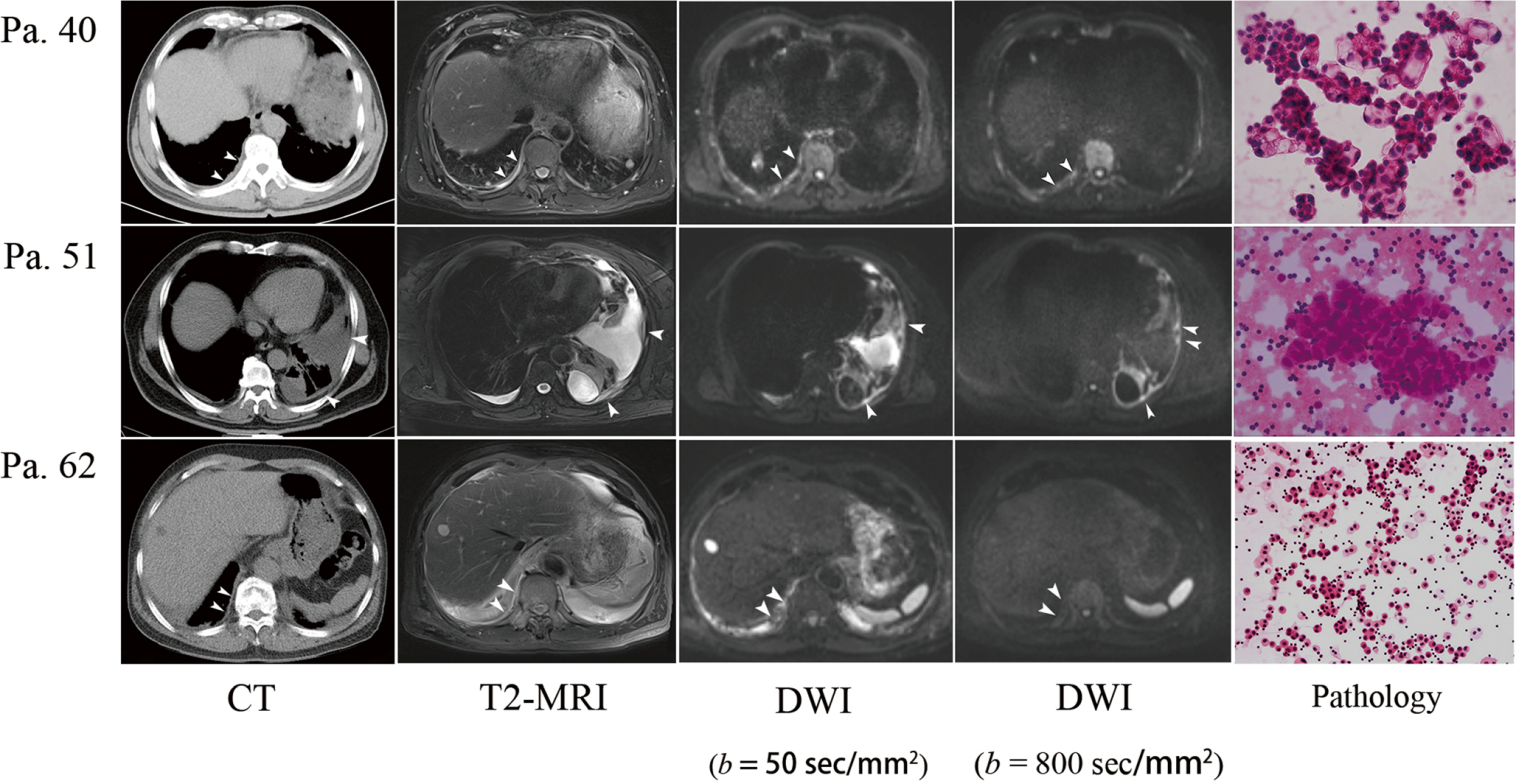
Representative cases. Patient 40 was a 60-year-old male diagnosed with primary adenocarcinoma. Circumferential pleural thickening was observed on CT and reported as PM. Similar features were observed on T2-weighted MRI. Multiple hyperintense areas were observed on DWI at a low *b* value (50 s/mm^2^) and a high *b* value (800 s/mm^2^), and definite malignancy was concluded. The cytological results confirmed adenocarcinoma. Patient 51 was a 63-year-old female diagnosed with advanced adenocarcinoma with bone and liver metastasis. CT was equivocal in the pleural assessment. Thready pleural thickening was observed on T2-weighted MRI. Multiple hyperintense areas were present on DWI, especially at a high *b* value (800 s/mm^2^), and definite malignancy was concluded. Cytological results confirmed adenocarcinoma. Patient 62 was a 67-year-old male with recurrent pleural effusion. Circumferential pleural thickening was observed on CT, and definite malignancy was concluded. However, hyperintense areas present on DWI at a low *b* value (50 s/mm^2^) were not present at a high *b* value (800 s/mm^2^); thus, the patient was classified as benign. Benign results were reported by cytology, which was repeated three times, and effusion was completely resolved after antibiotic therapy and 6 months of follow-up.
